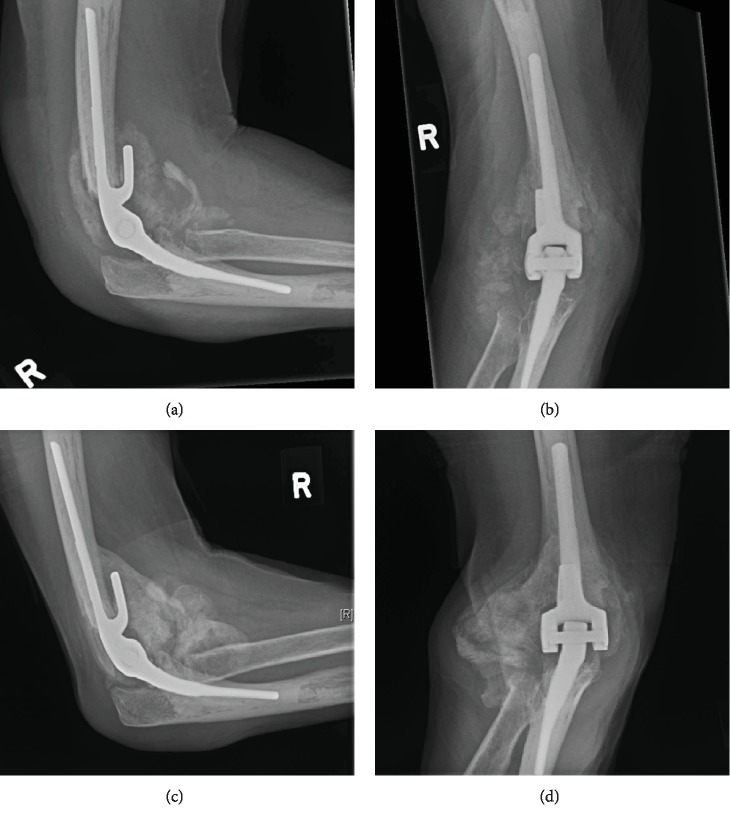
(a, b) Marked heterotopic ossification (HO) seen around the prosthesis at the 6-week follow-up X-rays. (c, d) Maturation of the HO seen at 18 weeks postoperation.
